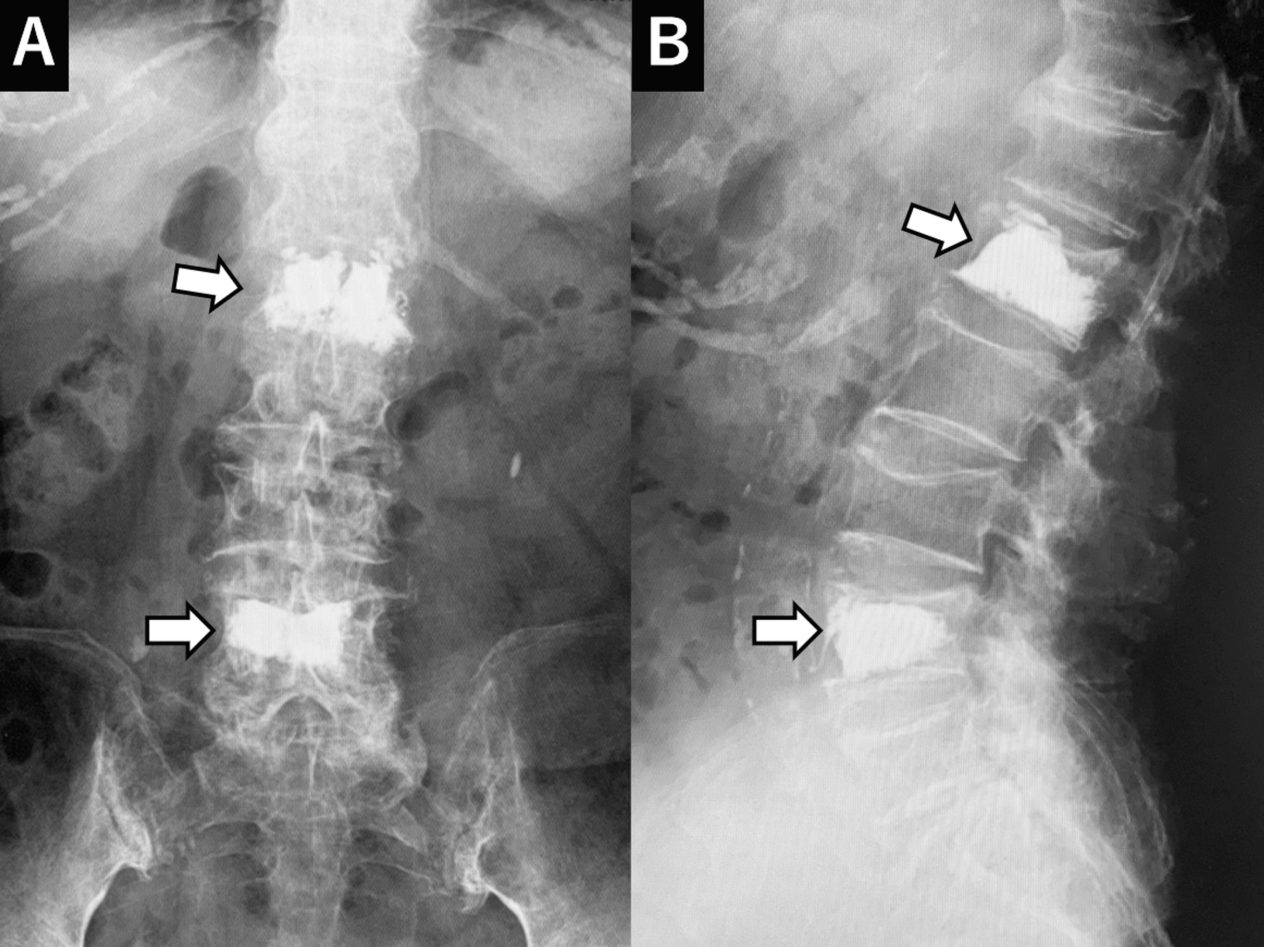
Post-first BKP X-ray An X-ray taken after the first balloon kyphoplasty (BKP) shows bone cement in L1 and L4 (arrows).
